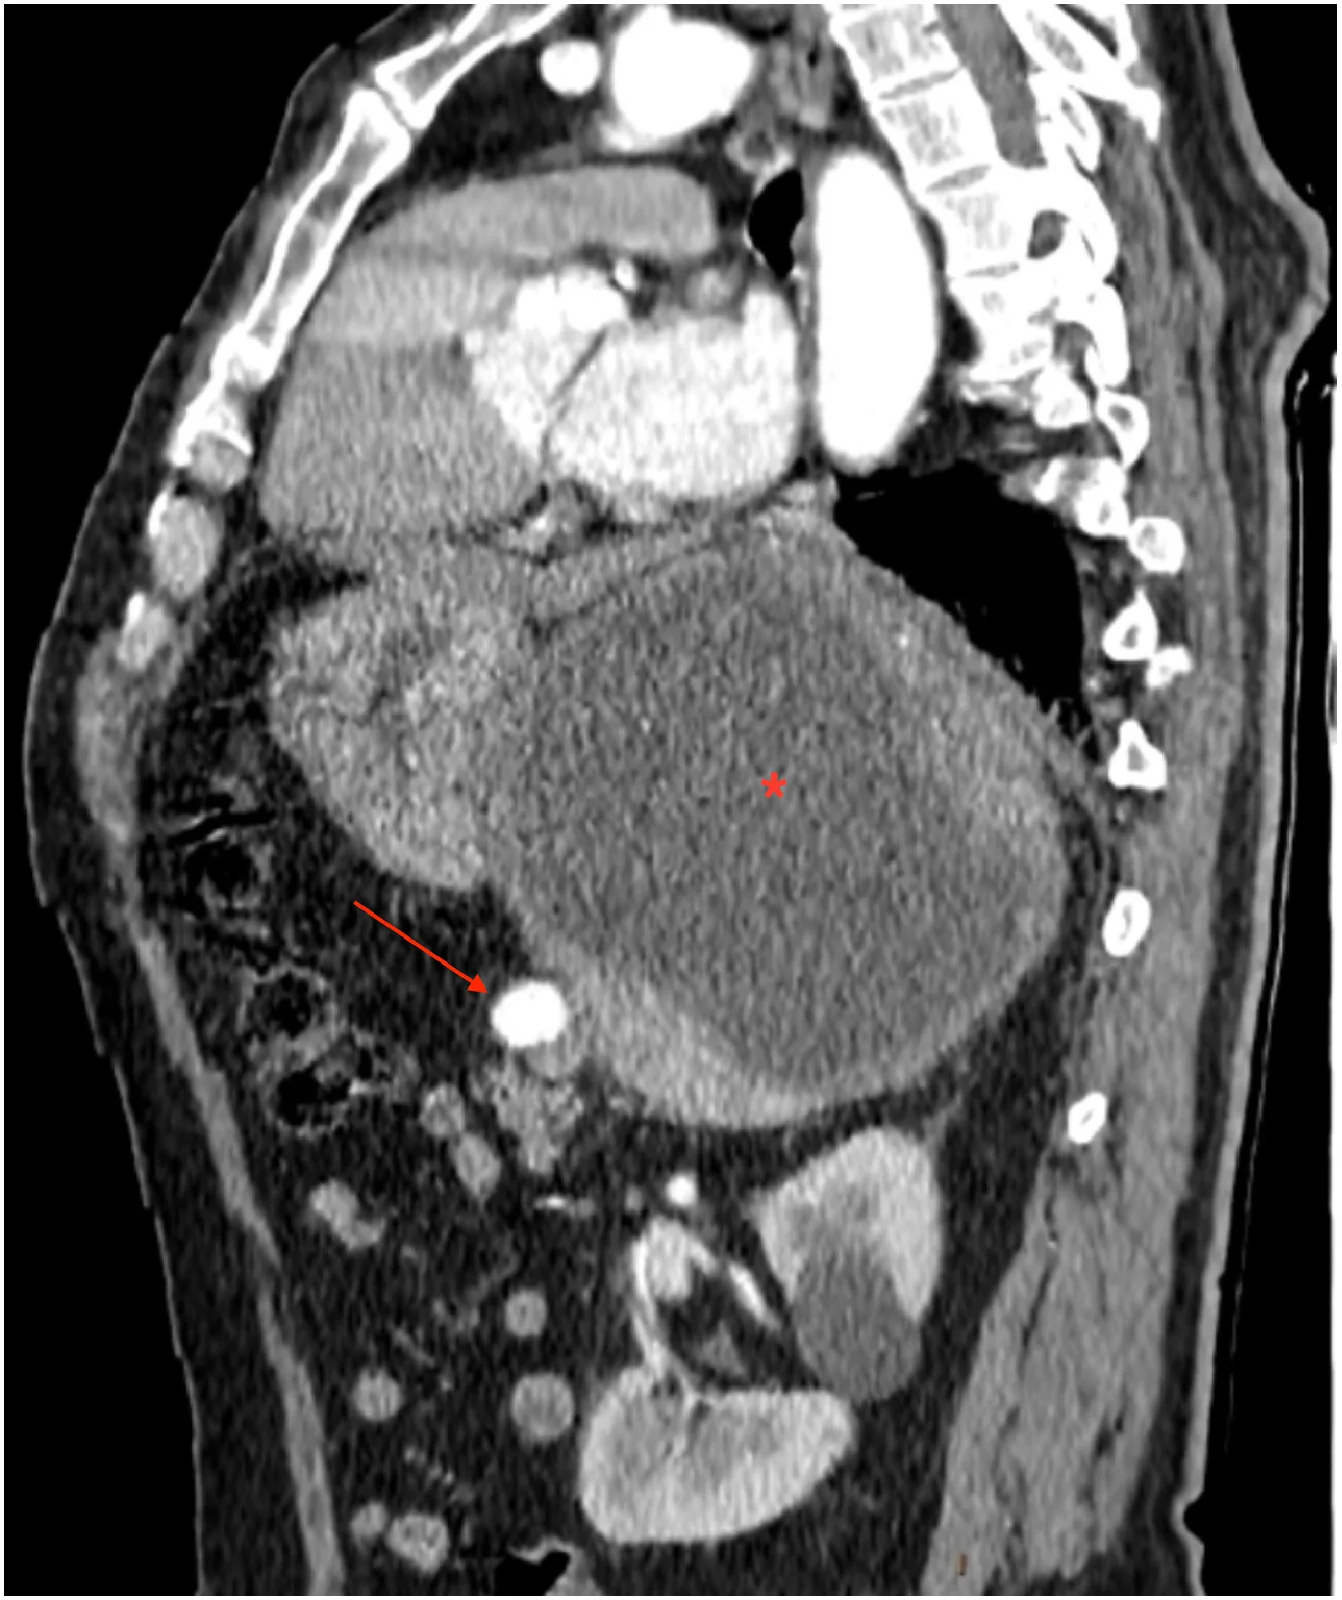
Sagittal contrast-enhanced CT reconstruction, arterial phase, showing the extent of the cystic splenic lesion (asterisk) with surrounding inflammatory changes. Arrow indicates vascular enhancement within the lesion, compatible with a pseudoaneurysm of the splenic artery.
